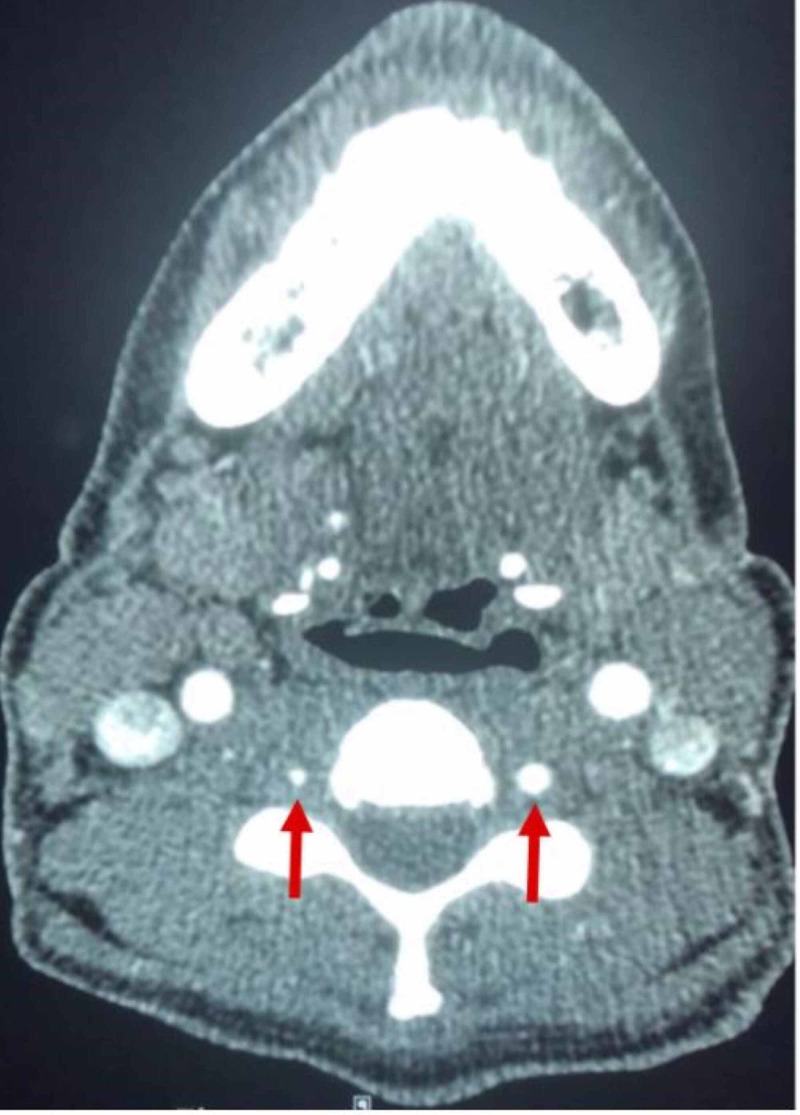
Vertebral Artery Narrowing Axial CT angiography (CTA) showing a narrowing of the vertebral artery lumen on the left than on the right (arrows).
